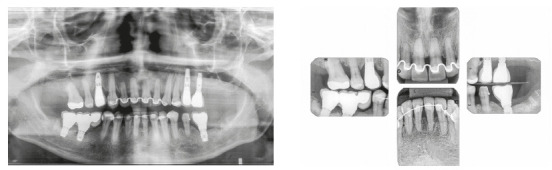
Final radiographs of Case 3.
